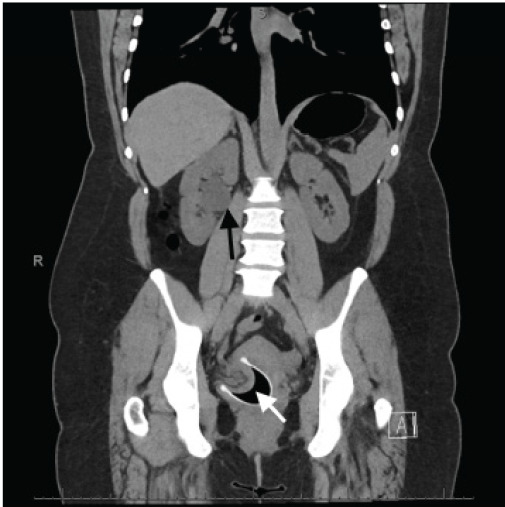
Computed tomography of the kidneys, ureters, and bladder showed mild right hydronephrosis (black arrow) with a transition point at the lower pelvis secondary to a malpositioned menstrual cup with parts of the right vaginal wall, ureter, and adnexal vasculature insinuating into the cup (white arrow).
